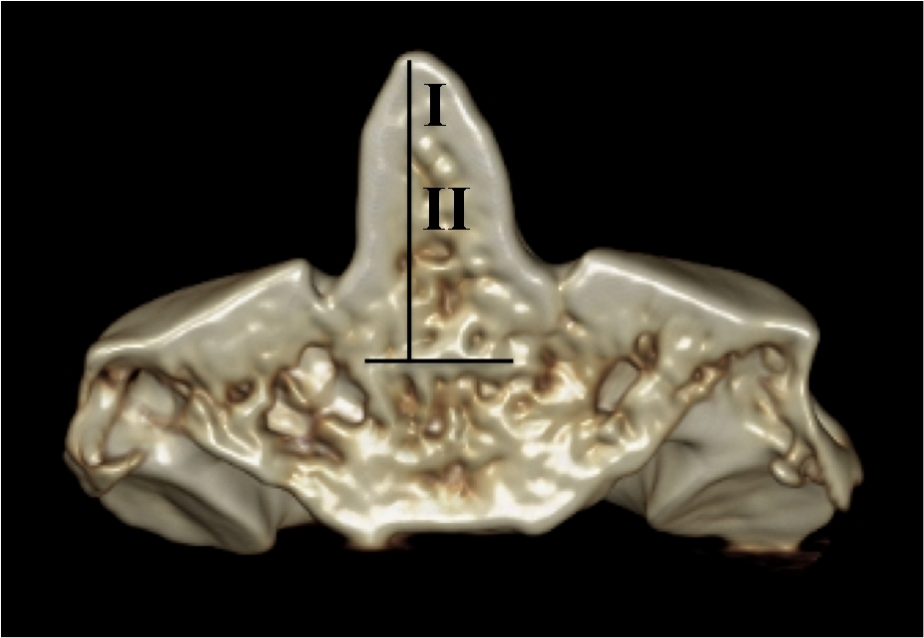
3D CT reconstruction of C2. Zones I and II are based on defining the dens (vertical line) as the midline portion of C2 that fuses with its body at the synchondrosis (horizontal line).
